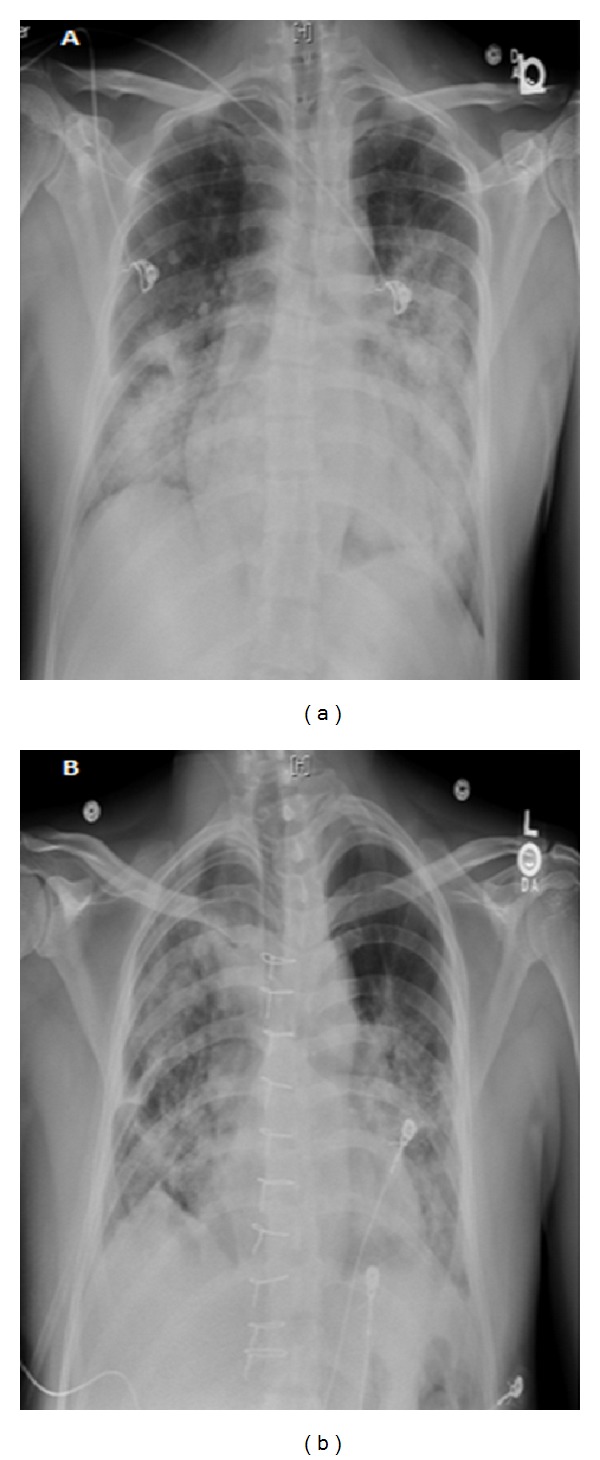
(a) Chest X-ray on admission day, (b) postoperative day 6.
